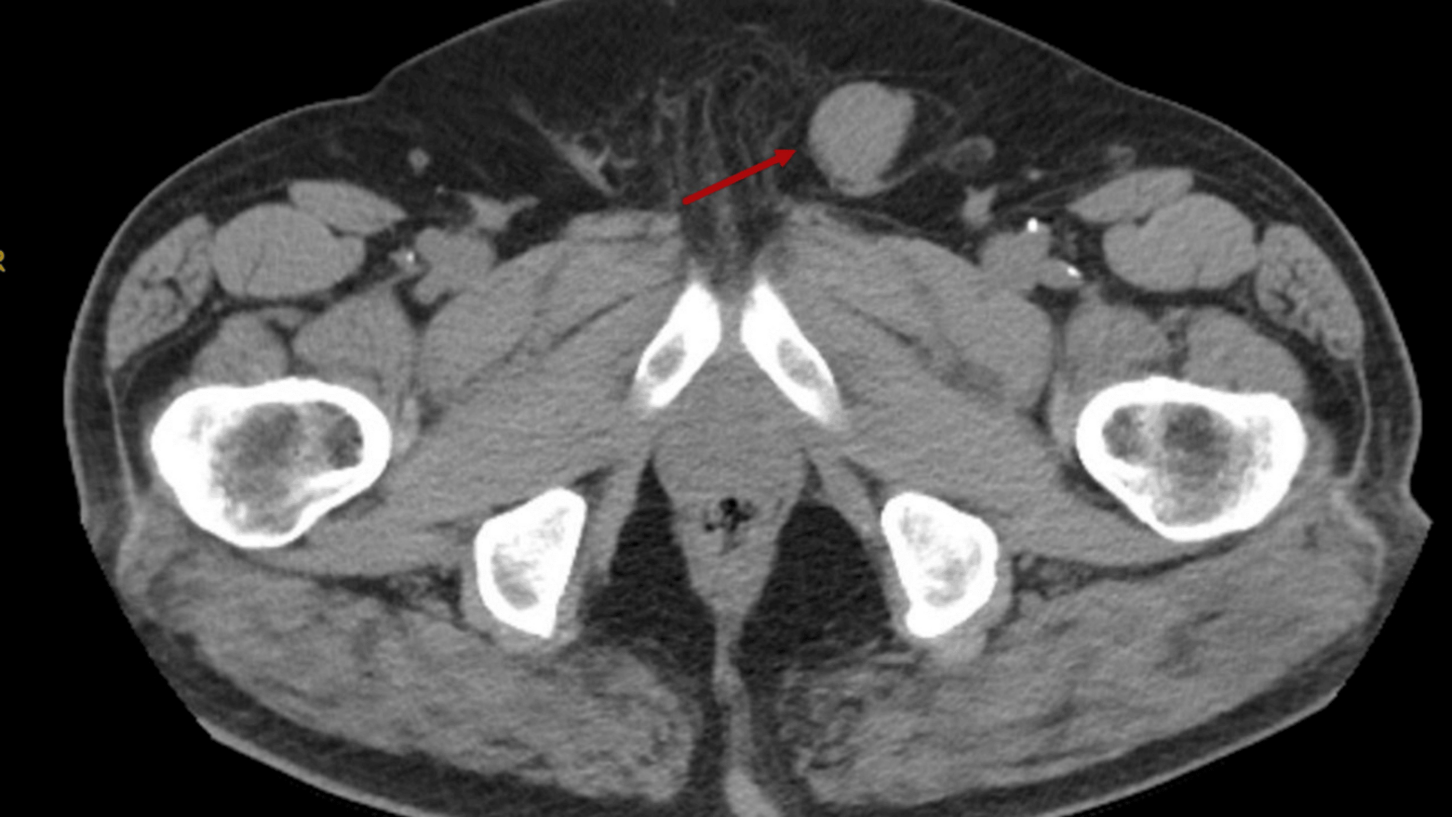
CT Abdomen and Pelvis A stable soft tissue density material is visualized within the left spermatic cord.
